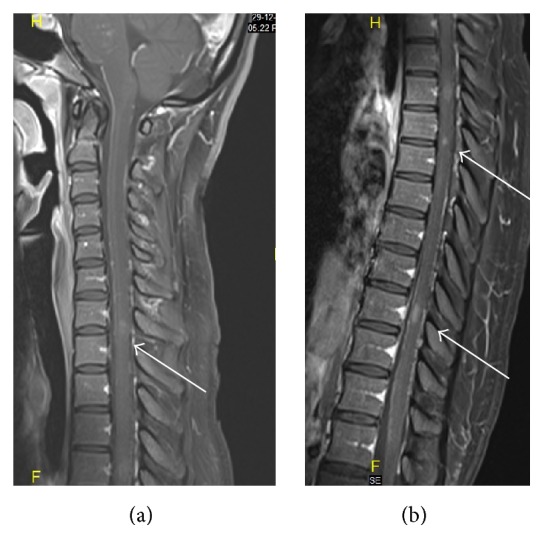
Sagittal T1 weighted MRI after IV gadolinium based contrast medium injection, of the spinal cord, showing multifocal punctate and linear enhancement at cervical and thoracolumbar levels (arrows in (a) and (b)), respectively.
